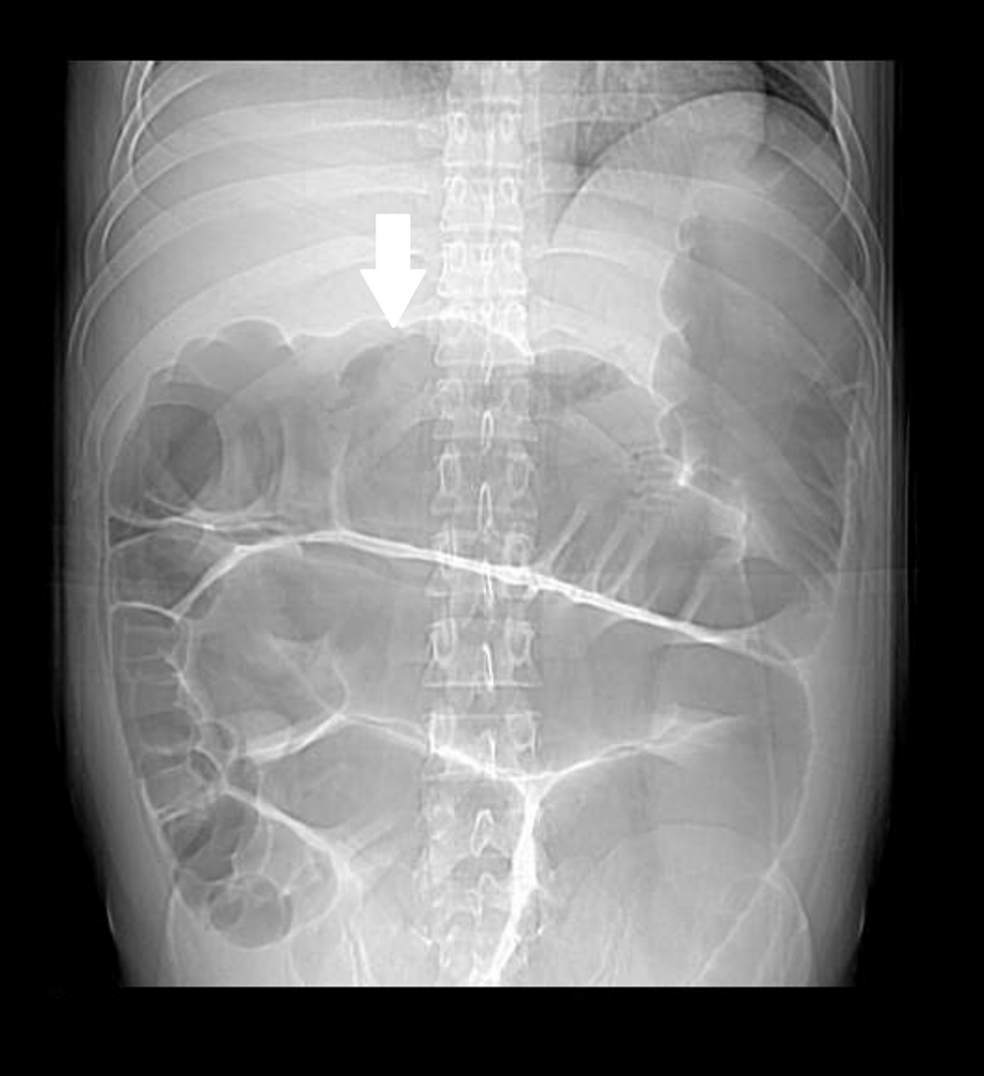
CT scan of the abdomen showing multiple distended small and large bowel loops.
